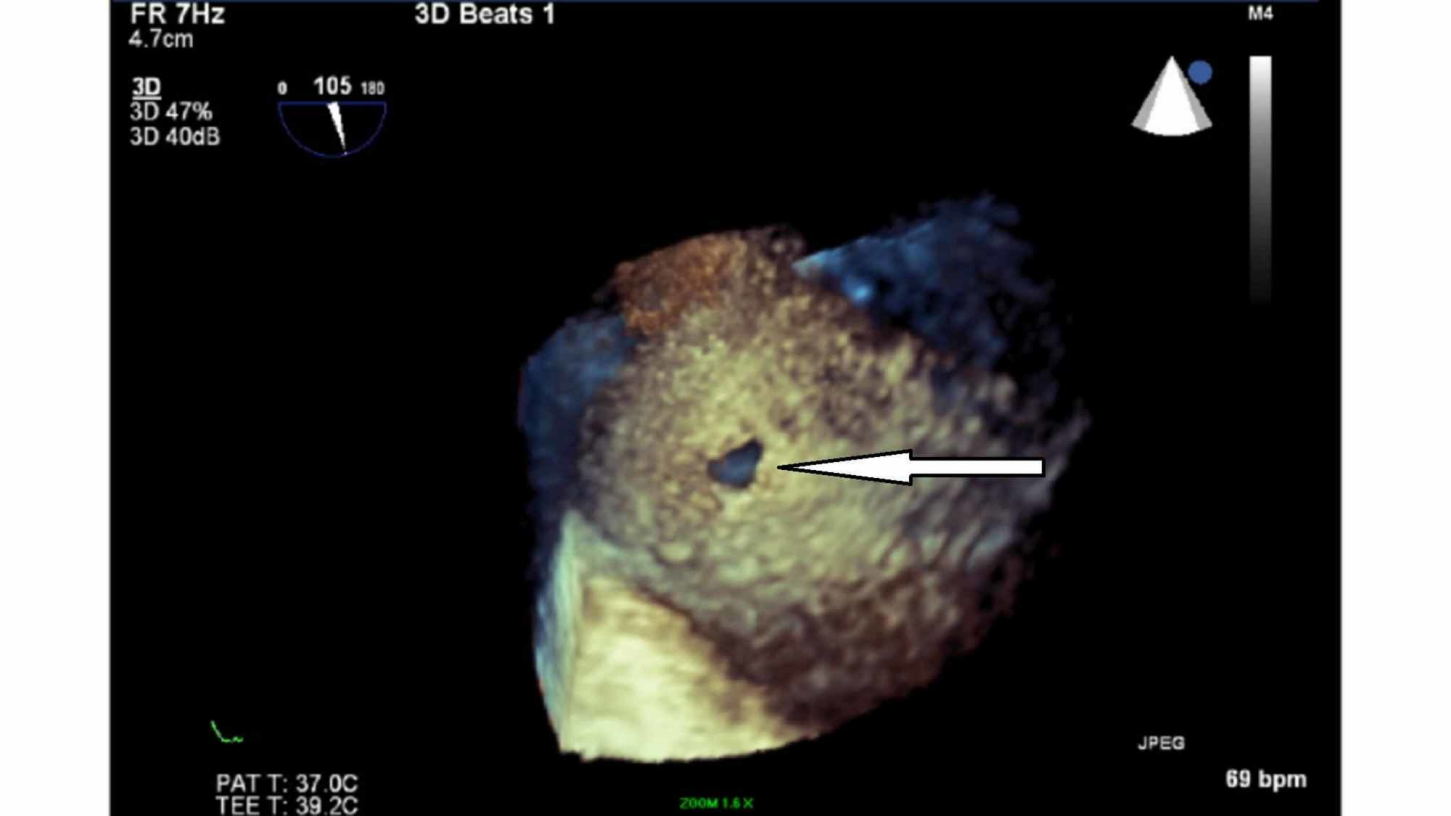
Real-time three-dimensional transesophageal echocardiography (RT-3D TEE) RT-3D TEE shows a posterior aortic tear (arrow).
